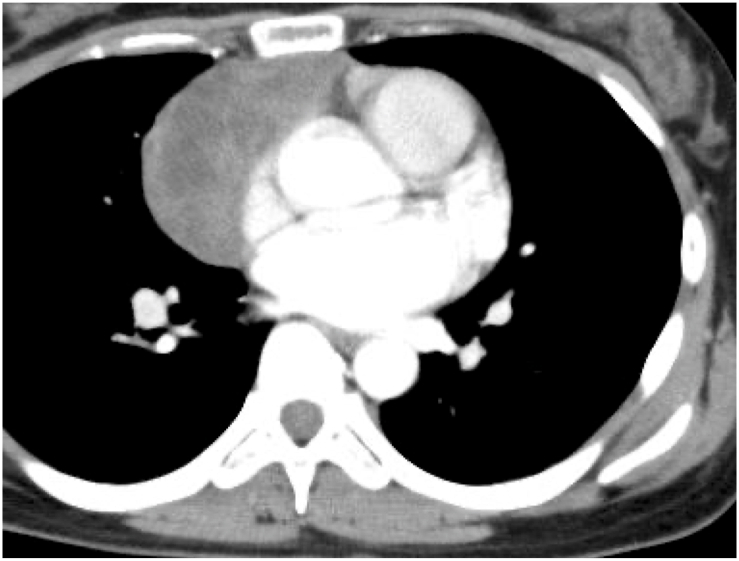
Chest CT image on initial presentation (10 years before the onset of Budd–Chiari syndrome) showing an anterior mediastinal tumor mass with a low-density area. The pathological diagnosis was invasive thymoma (type B2). She underwent systemic chemotherapy (CAMP therapy) followed by total thymectomy at that time. CT, computed tomography.
